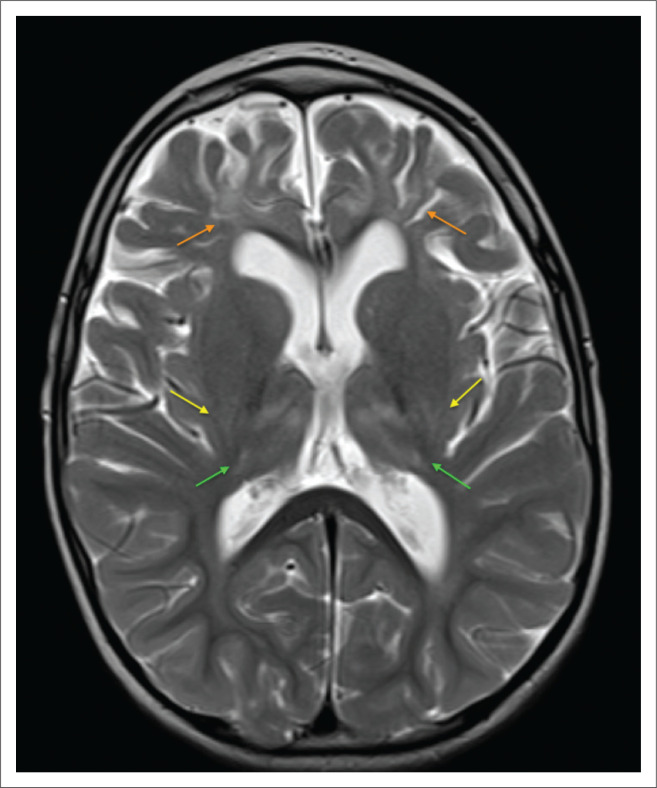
Axial T2-weighted image at the level of the basal nuclei performed on a 7-year-old male child. There is bifrontal encephalomalacia involving the middle and inferior frontal gyri (orange arrows) with ulegyria. Subtle hyperintensity is present at the posterior tips of the putamina (yellow arrows). Note in addition, loss of volume and more extensive hyperintensity of both thalami (green arrows). The combination is indicative of a mixed pattern of cerebral injury.
